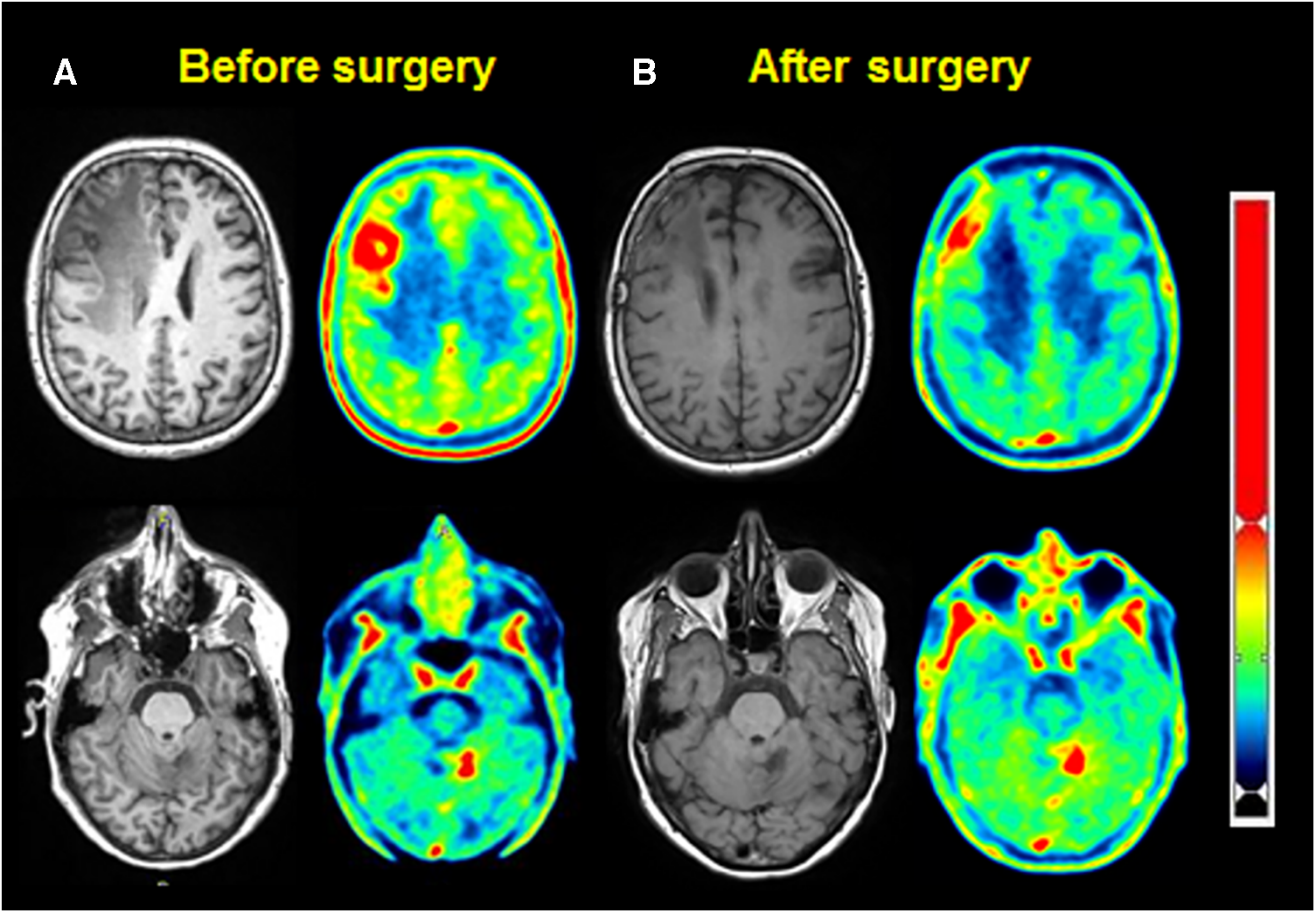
MRI (left) and [^18^F]FET PET (right), for frontal (up) and cerebellar (down) lesions, before (A) and after surgery (B).
